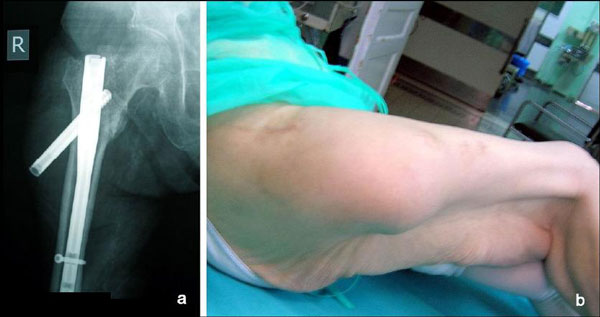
a 3 months post surgery anteroposterior radiographic view showing full lateral migration of the hip screw after a new fall; b Clinical view of the swollen right hip.
